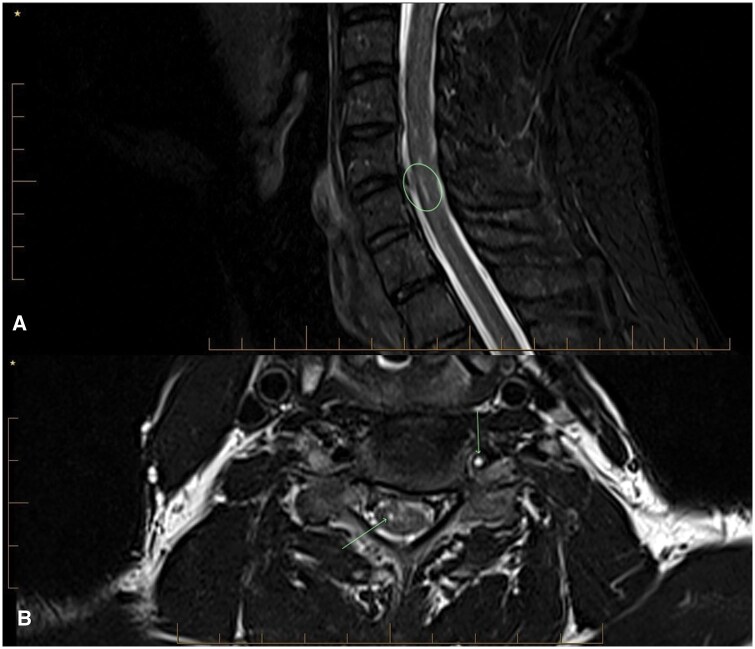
Follow-up imaging was completed 8 months post-index event. (A) MRI cervical spine Sagittal STIR image demonstrates linear T2 hyperintensity at C56 from prior right cord infarct. (B) MRI cervical spine Axial T2 image demonstrates T2 hyperintensity in the right central grey matter from a prior infarct. The left vertebral artery remains totally occluded with no flow signal.
